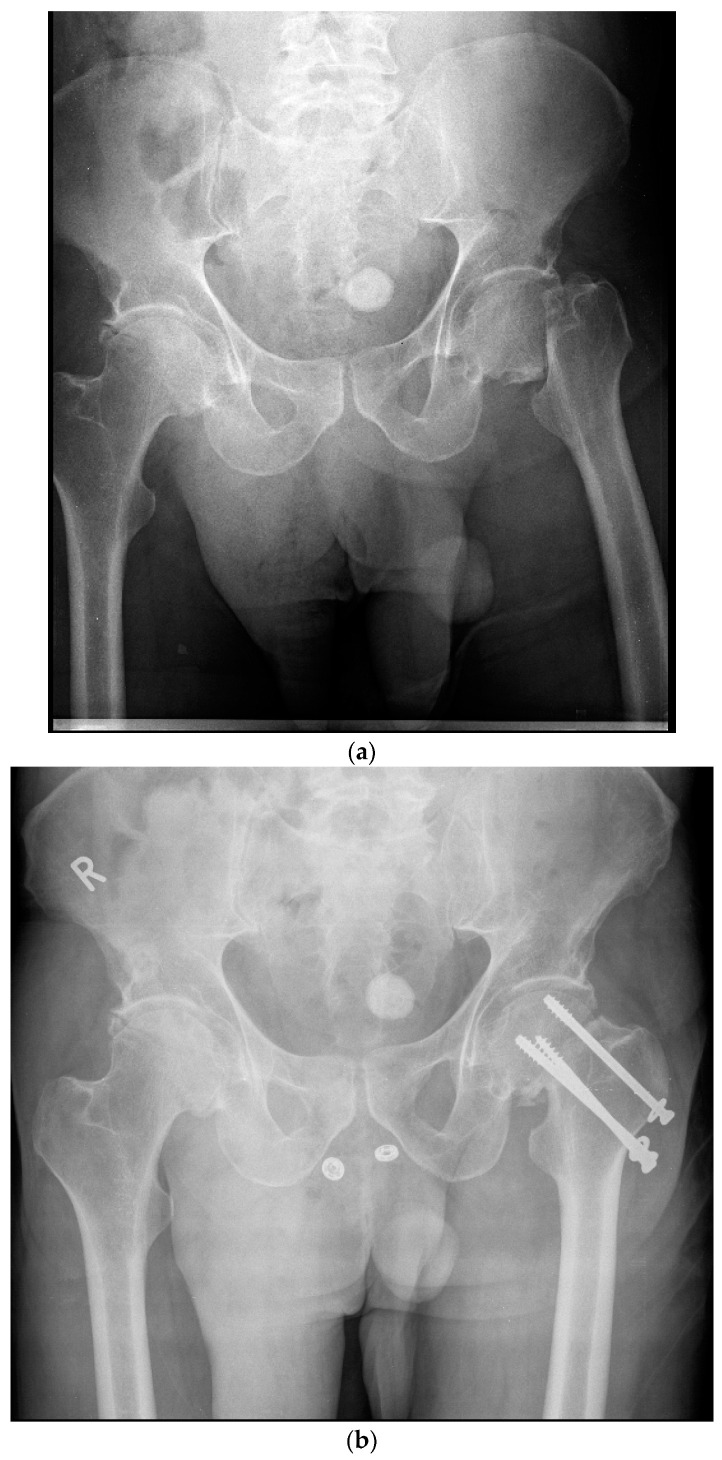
(a)—Pre-operative X-ray demonstrating a left sided displaced femoral neck in a 59-year-old male. (b)—Postoperative X-ray demonstrating operative fixation of the same fracture with cancellous screws. R—Patient’s right side.
